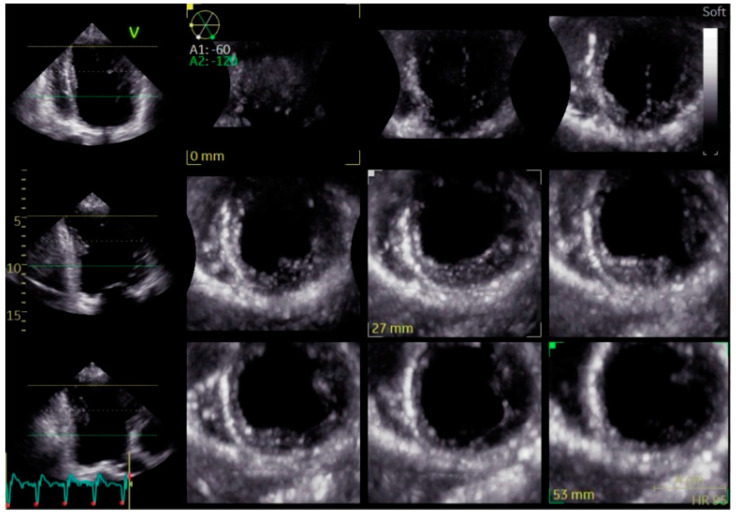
Three-dimensional, multi-slice transthoracic echocardiography showing short-axis views of the left ventricle at different levels. The images highlight increased echogenicity of the interventricular septum, suggestive of septal fibrosis, which may indicate myocardial remodeling.
